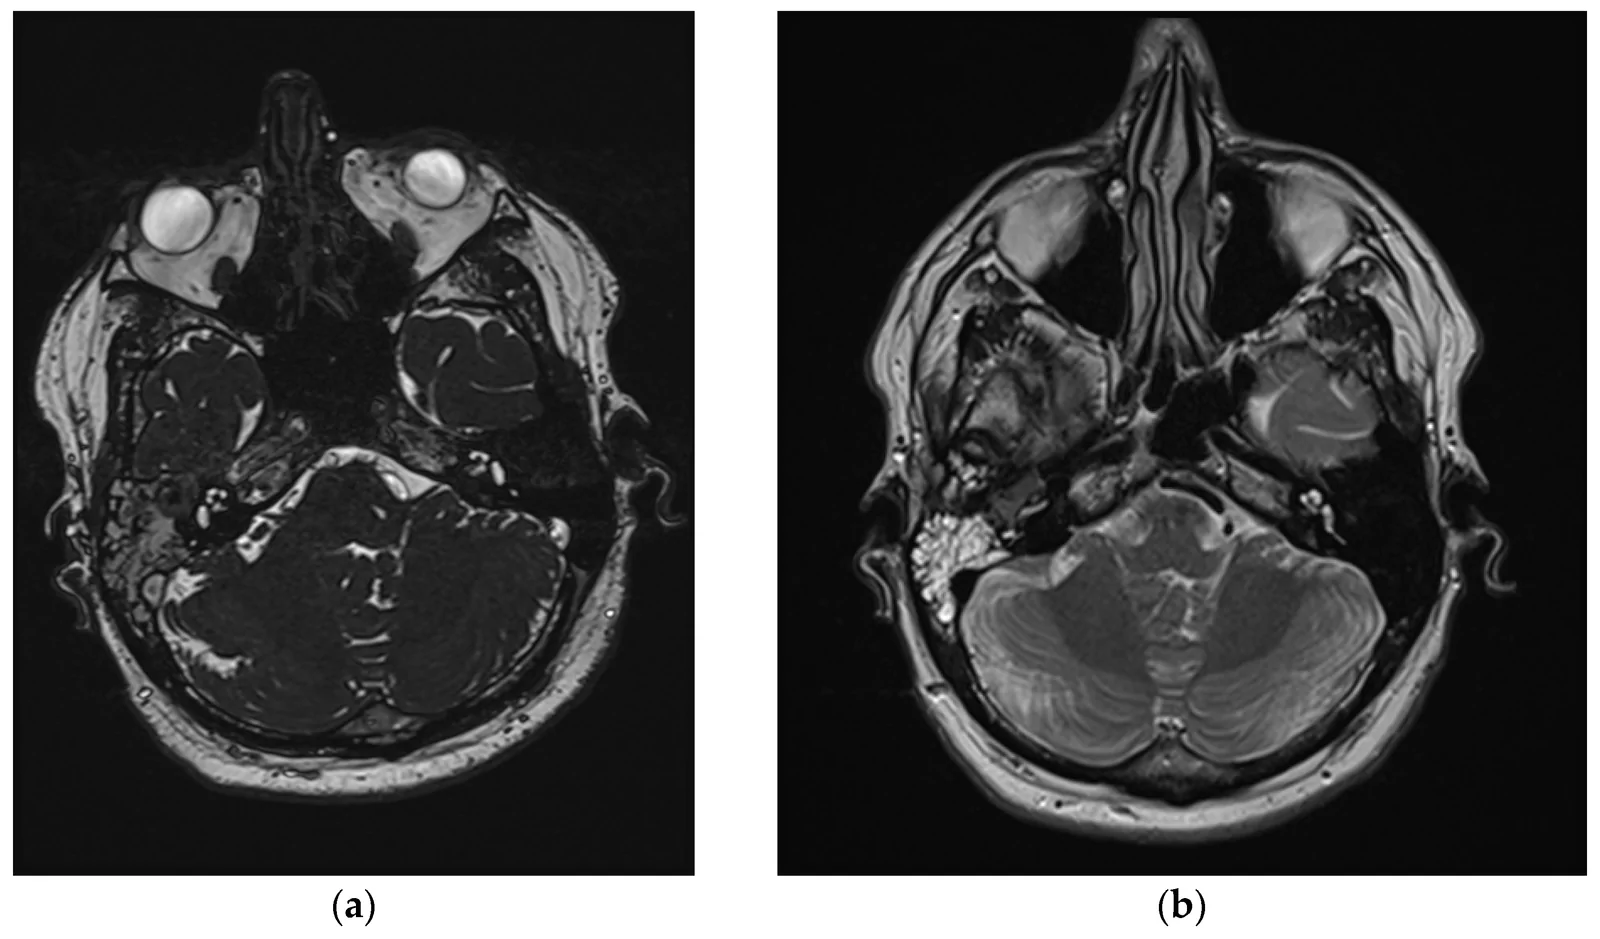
MRI scans of Patient 4 (J.P.): (a) T1-weighted sequence; (b) T2-weighted sequence.
